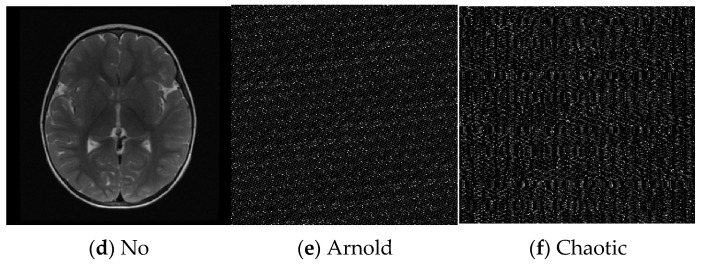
Encrypted MRI images for normal and tumor cases.
